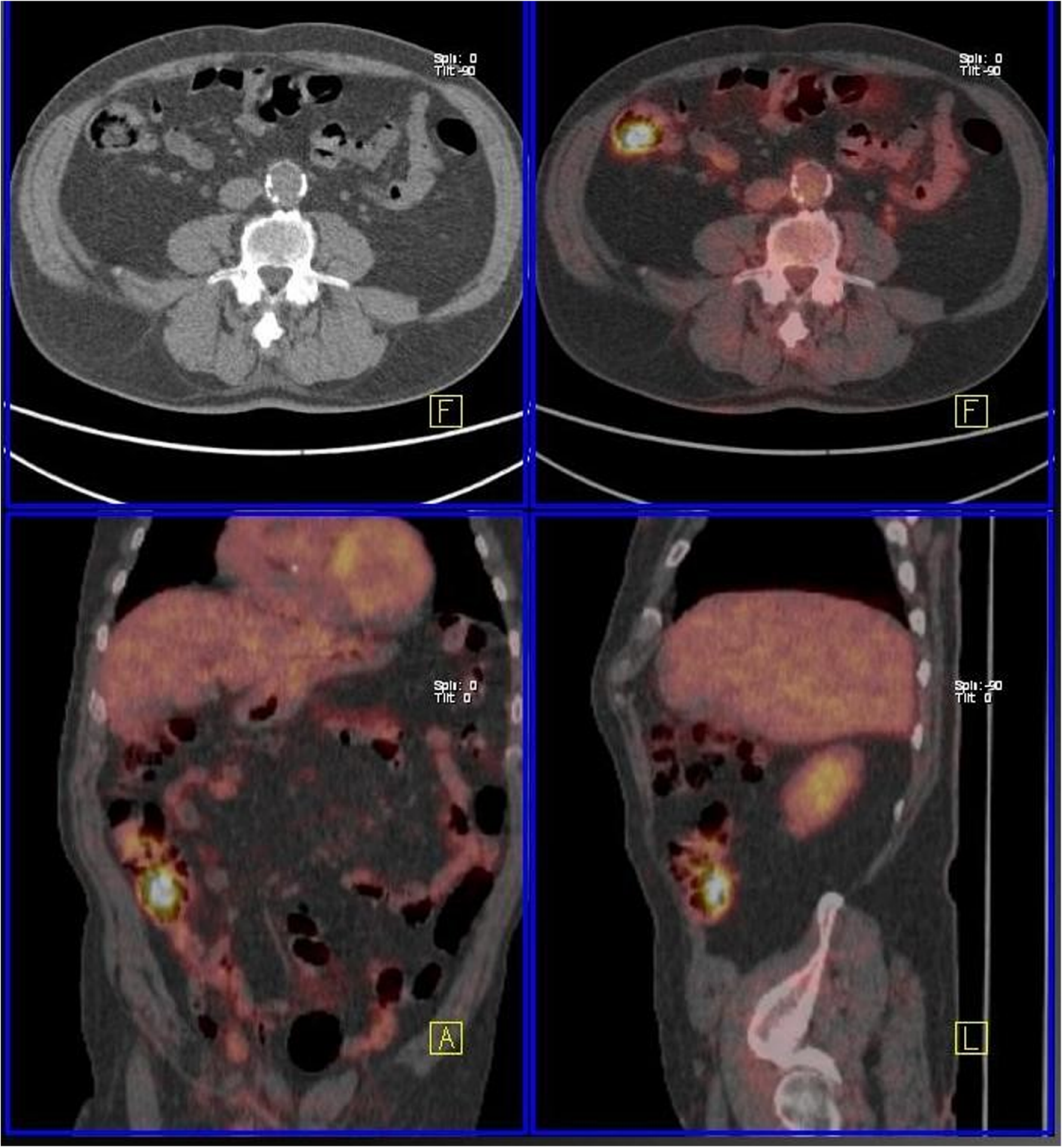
PET/CT incidental detection of cancer of the ascending colon associated with branch-type IPMN of the pancreatic head (PET negative)
